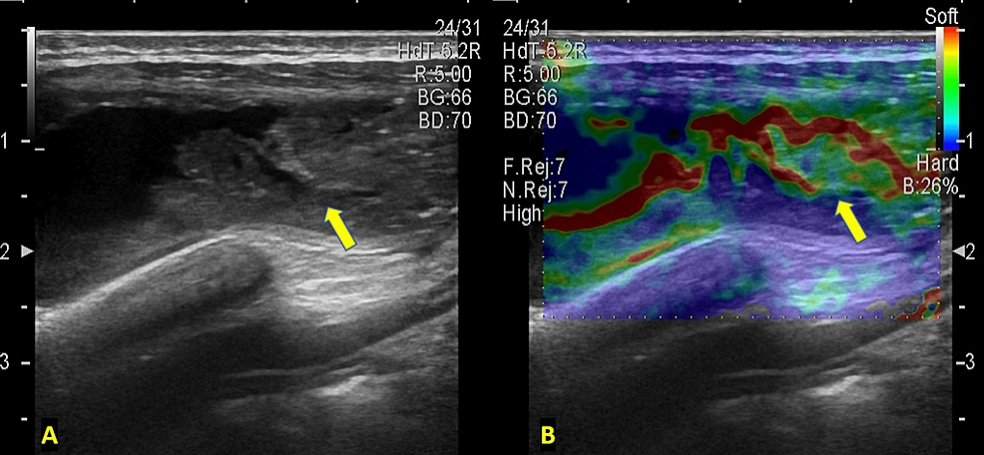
(A) B-mode ultrasound imaging showing a gray-scale image of the abscess (depicted by arrow) on the left. (B) The elastography image overlapped on the gray-scale image showing soft colors (depicted by arrow) red, yellow, and green with specks of aqua indicating softer consistency.
